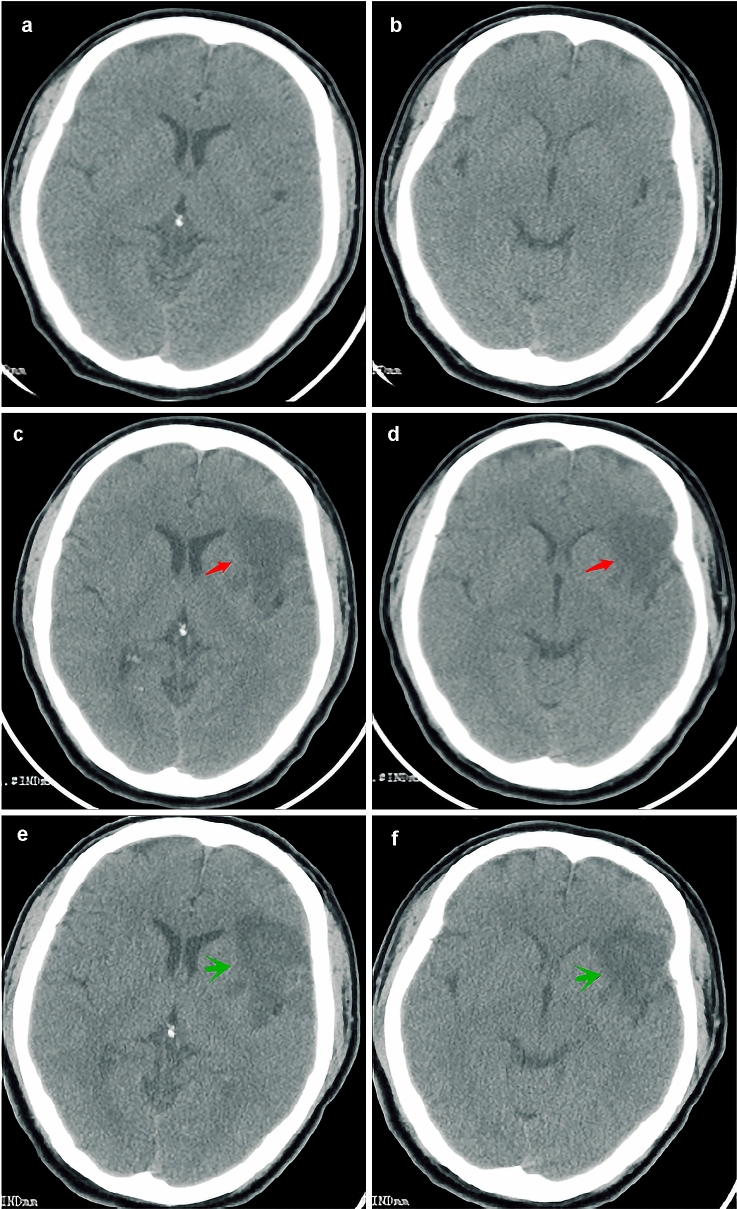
Emergency brain CT before intravenous thrombolysis showing no obvious abnormalities (a, b). Repeated brain CT showing an acute infarction in the frontotemporal area (arrow) and no hemorrhagic transformation at 35 h (c, d) and 1 week (e, f) after the onset. *CT* computed tomography
